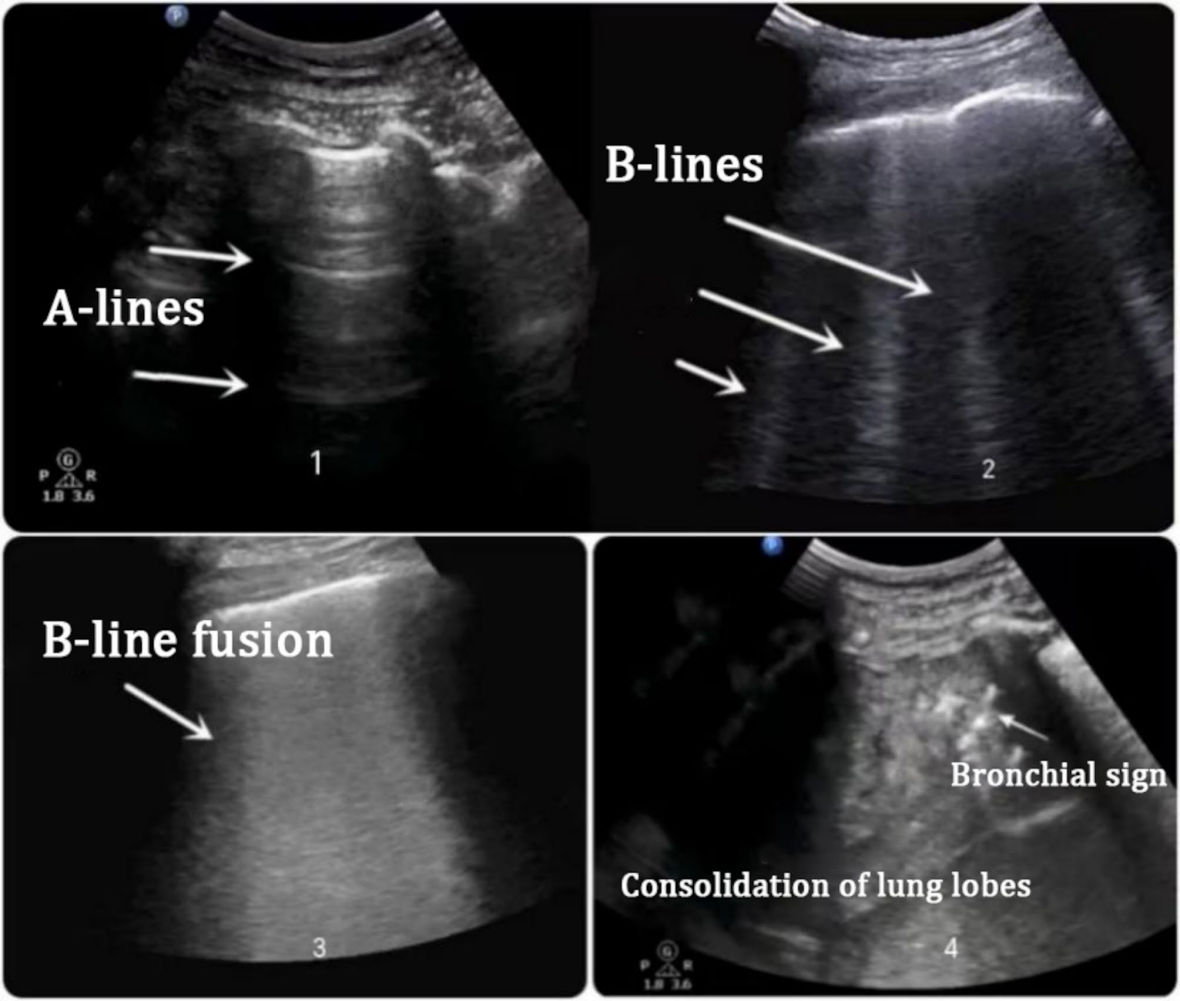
Lung Ultrasound Score (LUS) Chart. (1) A score of 0 indicates normal lung aeration (predominance of A-lines, indicative of healthy lung tissue). (2) A score of 1 signifies a moderate decrease in lung aeration (presence of 3 or more well-separated B-lines). (3) A score of 2 points to a severe reduction in lung aeration (diffuse confluent B-lines or minimal subpleural consolidation). (4) A score of 3 represents complete loss of lung aeration (liver-like appearance of lung tissue with or without air bronchograms)
